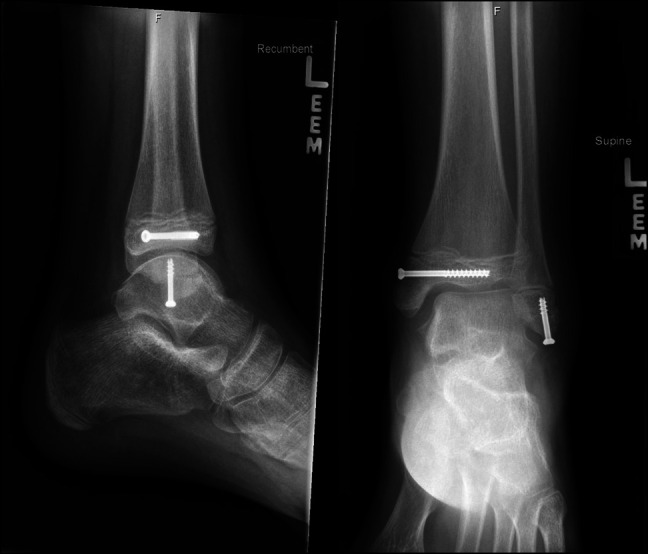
Postoperative AP and lateral view ankle radiographs after surgical fixation.
